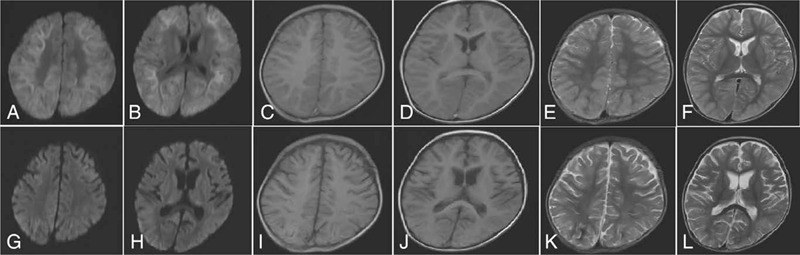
Radiological examination of a 17-month-old male patient with AESD. (A–F) Brain magnetic resonance imaging on the fourth day after onset revealed (A and B) reduced diffusion in the bilateral subcortical white matter on diffusion-weighted images. (C and D) The T1-weighted and (E and F) T2-weighted images were normal. (G and H) Brain MRI on day 14 showed the absence of reduced diffusion on diffusion-weighted images. (I and J) T1-weighted and (K and L) T2-weighted images showed cortical atrophy and focal necrosis.
